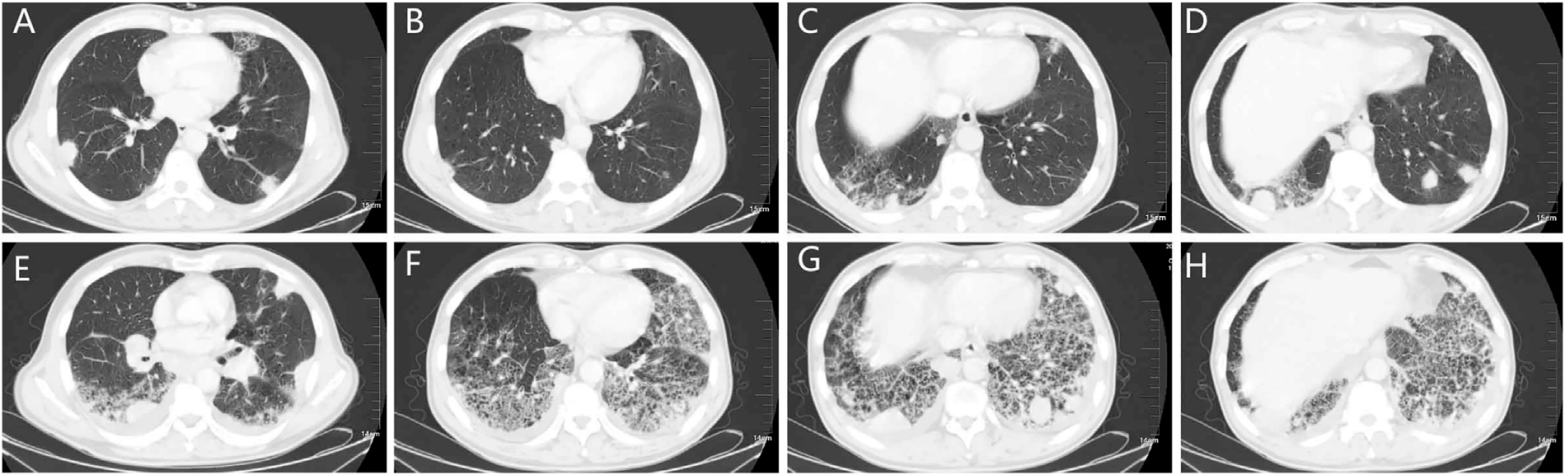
The chest CT of patient one. (A–D): chest CT showing the nodes in the lungs and the local grid change in the right lower lobe. (E–H): chest CT showing the pneumonitis 74 days after afatinib therapy, the pneumonitis manifested subpleural reticular shadow, ground glass and consolidation, and beehives, distributed in both lower lobe of both lungs. CT: computed tomography.
